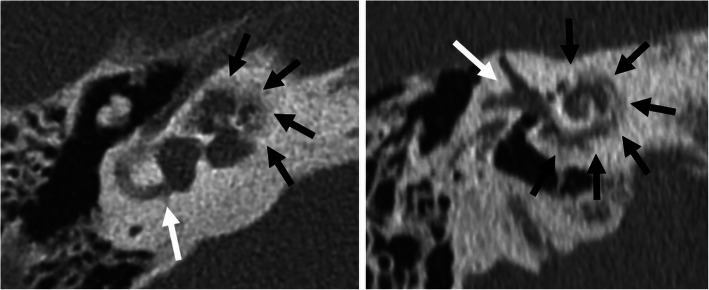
A 70-year-old male patient, with progressive SNHL from advanced otosclerosis. HRCT axial (left image) and paracoronal (right image) image show irregular ossifications affecting the cochlea (black arrows). Vestibulum and semicircular canals (white arrows) are not affected
